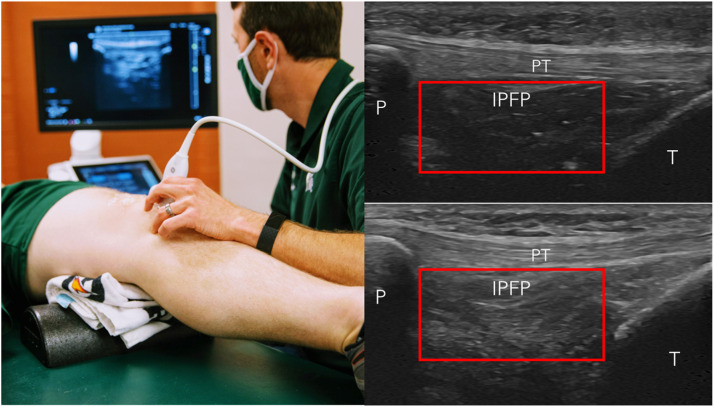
Anterior Knee Ultrasound Image Acquisition and Quantitative Assessment of Infrapatellar Fatpad Echo-intensity. Left Pane = patient and probe positioning to acquire images of the anterior knee. Right Panes = ultrasound image acquired in this patient and probe position to visualize the infrapatellar fatpad (IPFP) deep to the patellar tendon (PT), distal to the patella (P), and proximal to the tibia (T). Right top picture shows an example image of a fatpad without MRI-confirmed Hoffa-synovitis and the Right Bottom picture shows the same patient's contralateral knee with MRI-confirmed Hoffa-synovitis. The red rectangle in both pictures represents the borders of the region of interest used to quantify the IPFP signal intensity. The region of interest rectangle is standardized at a height of 10 millimeters, but the width was adjusted to fit each participant's knee as the largest width that fit within the IPFP while not including the overlying patellar tendon, the proximal patella, the distal tibia.
